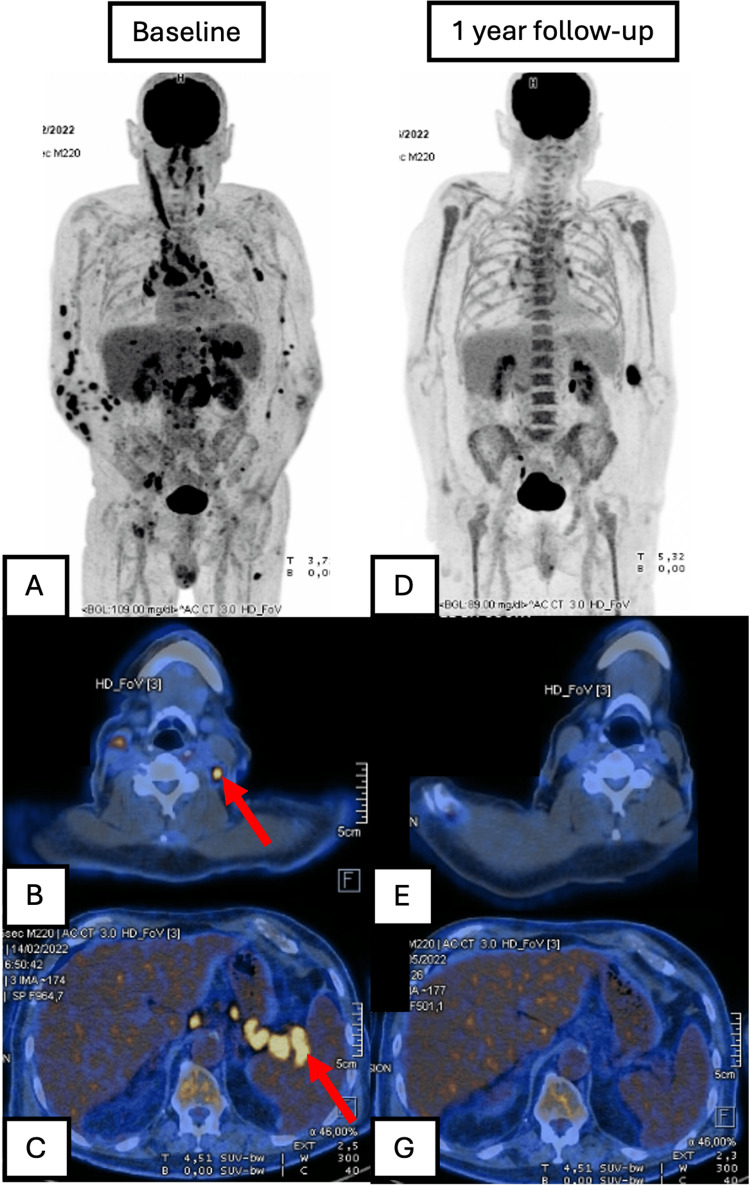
Baseline and 12‑month follow‑up FDG-PET-CT demonstrating complete metabolic response. (A) Whole‑body PET-CT scan at diagnosis reveals widespread hypermetabolic lymphadenopathy. (B) Axial PET-CT at the cervical level shows a left lateral cervical node (straight white arrow). (C) Coronal PET-CT below the diaphragm depicts multiple infra‑diaphragmatic adenopathies (straight white arrows). (D-F) Corresponding views obtained 12 months after six cycles of R‑CHOP: whole‑body (D), cervical (E), and infra‑diaphragmatic (F) images demonstrate complete metabolic response. FDG, fluorodeoxyglucose; R-CHOP, rituximab, cyclophosphamide, hydroxydaunorubicin, vincristine (Oncovin), and prednisone
